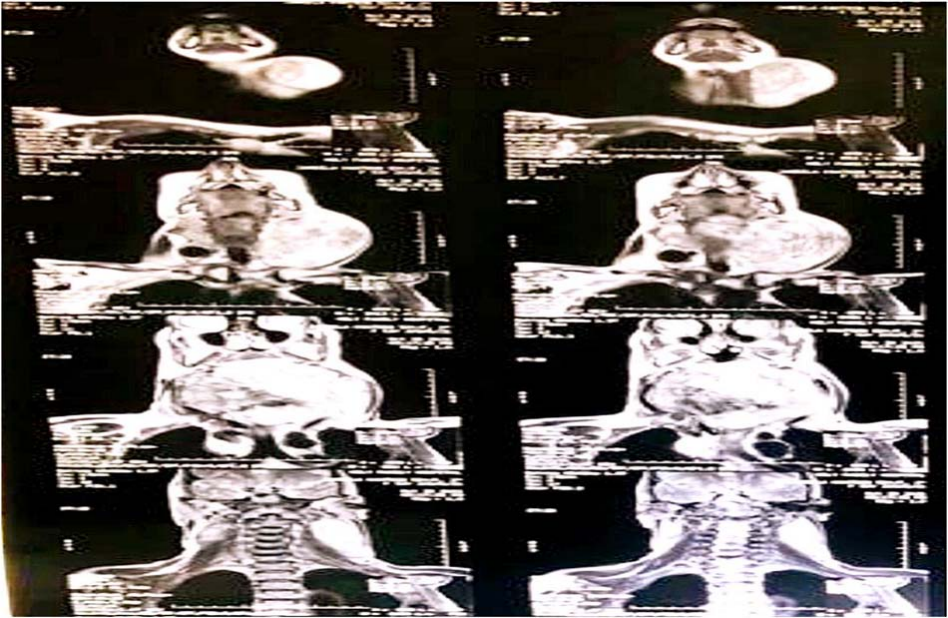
CT scan demonstration a well-defined mass in retropharyngeal space measuring 9 × 15 × 11 cm in various dimensions and chunk of calcific foci occupying most of the left retropharyngeal space.
